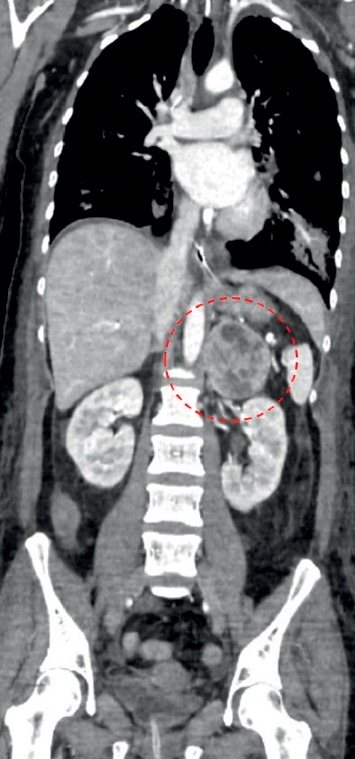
Contrast-enhanced total body CT scan revealing a left adrenal heterogeneous mass of 59 × 56 mm (red dots).
